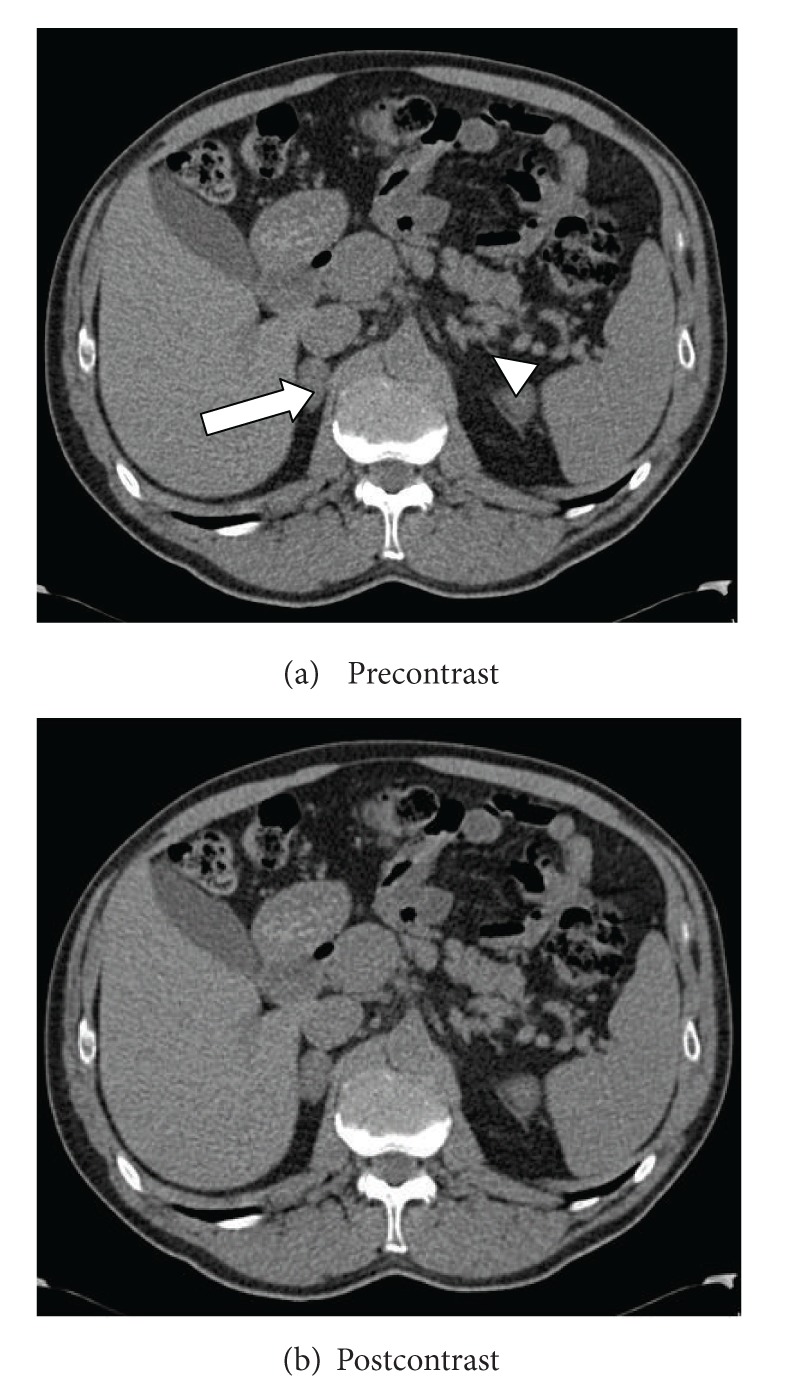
(a) Adrenal CT without contrast demonstrating a reduction in the size and the attenuation value of right adrenal mass (thick arrow); normal left adrenal gland (arrow head); (b) postcontrast image reveal no enhancement.
